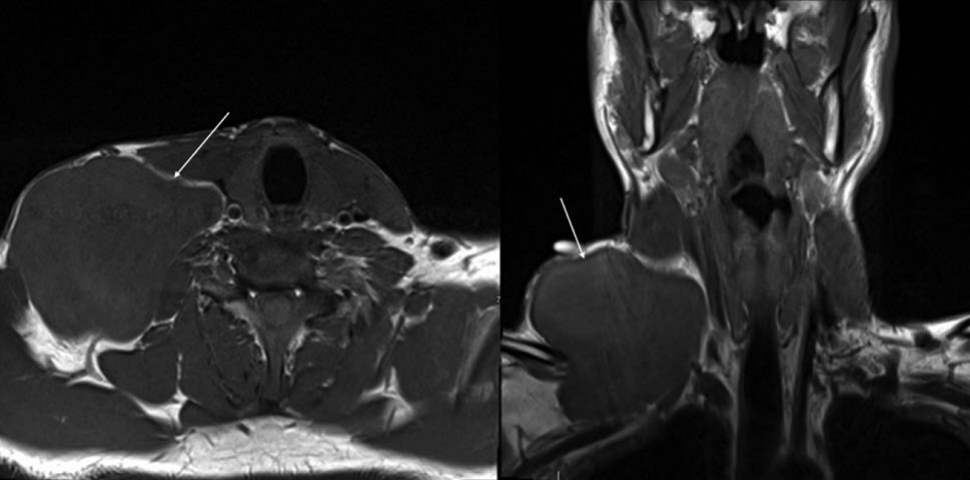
MRI axial and coronal slices demonstrating a large well-circumscribed right cystic mass, postero-lateral to the sternocleidomastoid, and in close proximity to the carotid trunk
